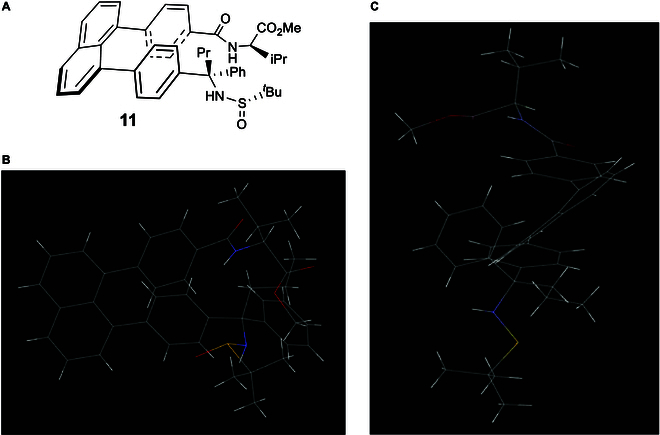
Staircase isomer 11 and its x-ray structural analysis. (A) Chemical structure of one staircase isomer. (B) View of its x-ray structure from top to bottom. (C) View of its x-ray structures from side to side.
